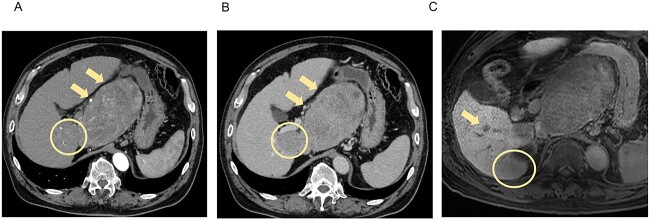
CT and MRI at the initial examination. Axial enhanced CT reveals mass lesions of 14 cm (A, B, arrow) and 3 cm (A, B, circle) in S1, with heterogenous enhancement in the arterial phase (A) and a washout pattern in the portal phase (B). Axial enhanced MRI shows the lesions in S1 and other lesions of 5 cm in S6/7 (C, circle) and 1 cm in S6 (C, arrow), which demonstrate decreased ethoxybenzyl uptake. S, segment.
